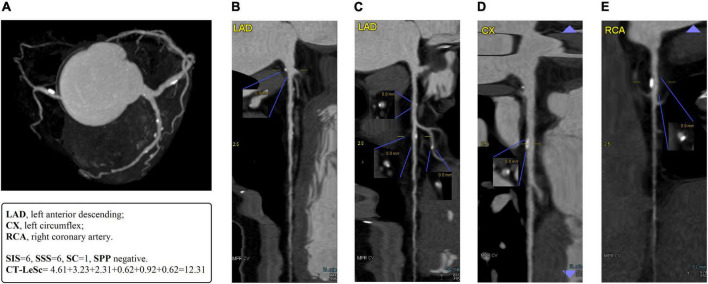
A case example of 55-years-old man with T2DM for 10 years. (A) The MIP of coronary tree with blood pool removal shows right dominance and six non-obstructive stenosis segments in LAD, D1, LCx, and RCA. (B) The straightened MPR of LAD shows a mixed plaque with mild lumen stenosis in LM. The CT-LeSc = 5 × 1.5 × 0.615 (weighting for localization × type of plaque × stenosis severity) = 4.61. (C) The straightened MPR of LAD shows mixed plaques with mild lumen stenosis in proximal and mid LAD, a calcified plaque with mild lumen stenosis in D1. The CT-LeSc = 3.23 (3.5 × 1.5 × 0.615), 2.31 (2.5 × 1.5 × 0.615), 0.62 (1 × 1 × 0.615), respectively. (D) The straightened MPR of LCx show a calcified plaque with mild lumen stenosis in proximal LCx. The CT-LeSc = 0.92 (1.5 × 1 × 0.615). (E) The straightened MPR of RCA show a calcified plaque with mild lumen stenosis in proximal RCA. The CT-LeSc = 0.62 (1 × 1 × 0.615). The total CT-LeSc of this patient = 4.61 + 3.23 + 2.31 + 0.62 + 0.92 + 0.62 = 12.31. There are six segment coronary plaques, so the SIS of this patient is 6. Mild lumen stenosis is found in all 6 plaques with score 1 of SSS, the SSS of this patient is 6. The SC = SSS÷SIS = 1. There is no severe proximal plaque in the proximal of LAD, LCx, and RCA, so the SPP is negative. MIP, maximum density projection; MPR, multiplanar reconstruction; LAD, left anterior descending; LM, left main; D1, 1st diagonal; LCx, left circumflex; RCA, right coronary artery; CT-LeSc, CCTA-adapted Leaman score; SIS, segment involvement score; SSS, segment stenosis score; SC, stenosis coefficient; SPP, severe proximal plaque.
